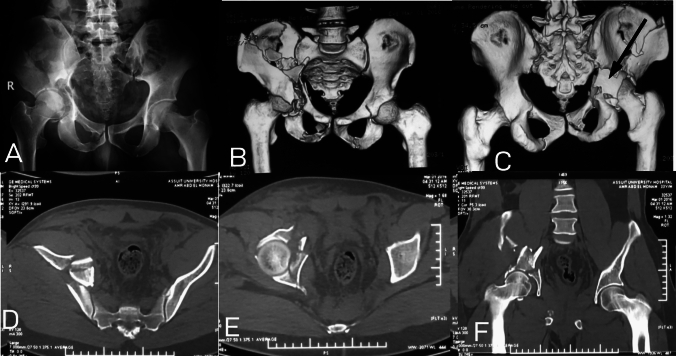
Both columns with posterior wall; A–F AP view and CT scan showing both columns fracture associated with posterior wall (arrow). The posterior wall in this case is the extended variant as described by Letournel
